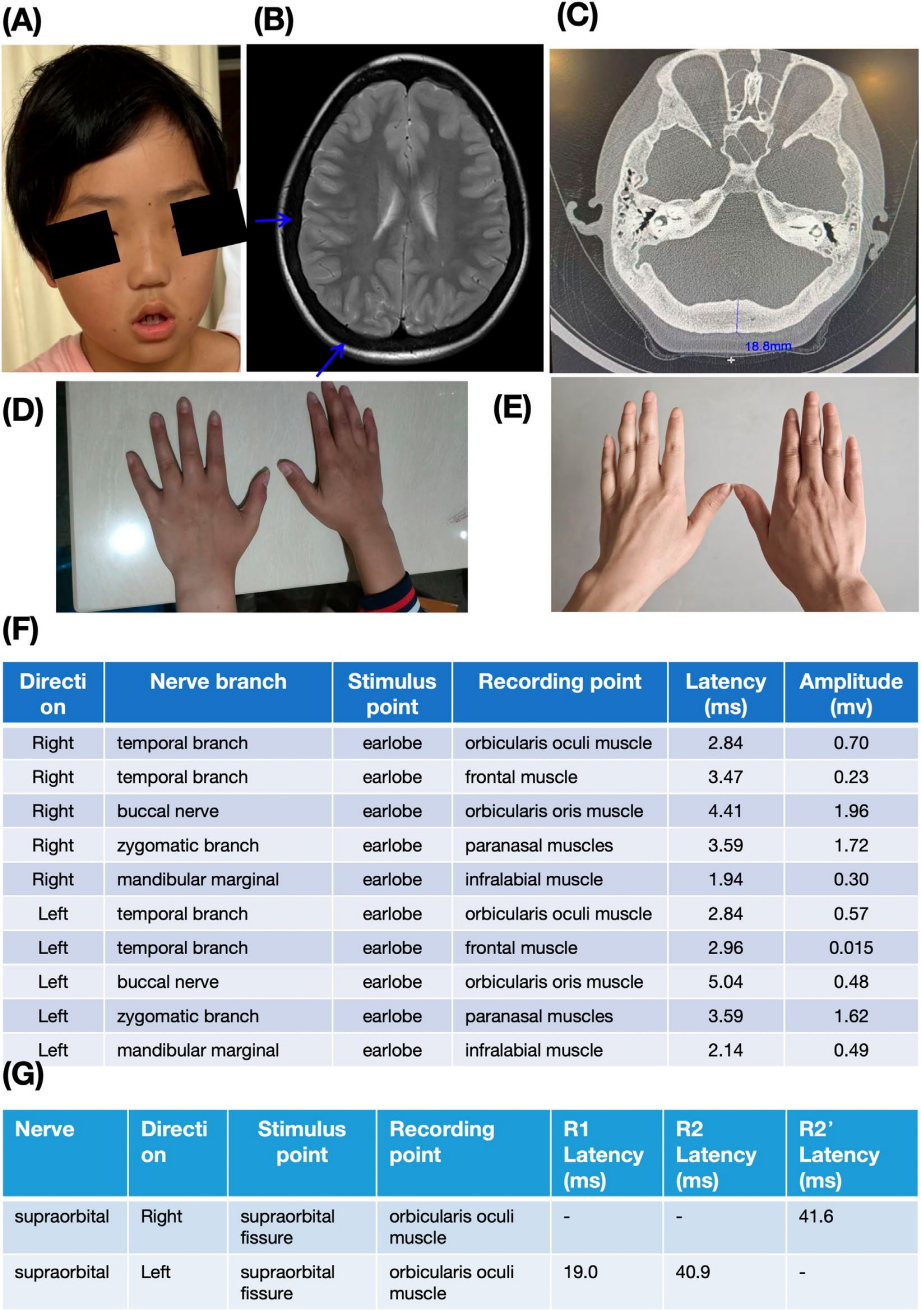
Clinical features of *SOST* genetic variant. (A) Facial photographs of the proband who exhibited abnormal facial expressions with numbness. (B) The brain MRI indicates that the skull thickens and the signal of the diplopia disappears (blue arrow). (C) Temporal bone CT results indicate diffuse osteosclerosis, involving bilateral ossicular chains and internal auditory meatus, and bilateral internal auditory meatus stenosis. (D) The hands of the proband. (E) The hands of the sister. (F) Measurement of motor afferent function of the facial nerve. (G) Measurement of blink reflex.
